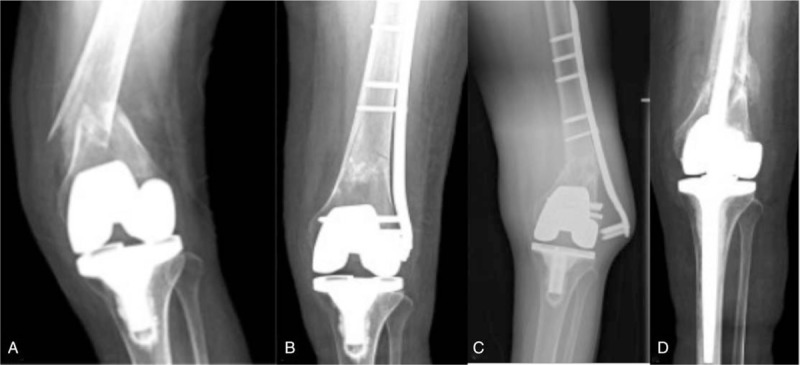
Anteroposterior radiographs of case 1, sustained a left Rorabeck type II closed distal femoral fracture (A), internal fixation of LISS plate was performed immediately (B). 7 months later, screws of LISS plate were broken and fracture displacement occurred again (C), underwent total knee arthroplasty revision surgery with rotating hinged knee prosthesis finally (D).
